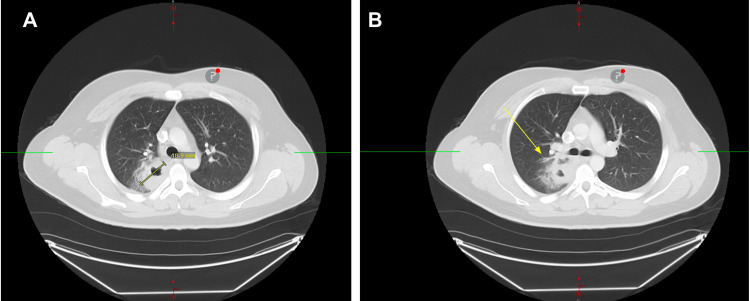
CT of the chest with contrast (A, B) CT chest with contrast performed showing a thick-walled irregular cavitary lesion in the posterior medial right upper lobe posterior to the right hilum measuring approximately 4.6 x 4.8 x 4.5 cm. The right hilar lymph nodes are mildly enlarged, and there is adjacent inflammatory change. Lungs are otherwise clear without any pleural effusion or pneumothorax. CT, computed tomography
